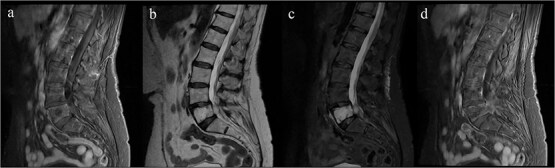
Preoperative MRI showing a L5 vertebral body lesion with extension to the left foramina and radicular obliteration. (a) Sagittal T1- weighted sequence with hypointense signal. (b) T2 and STIR hyperintense signal. (c) Minimum gadolinium enhancement.
